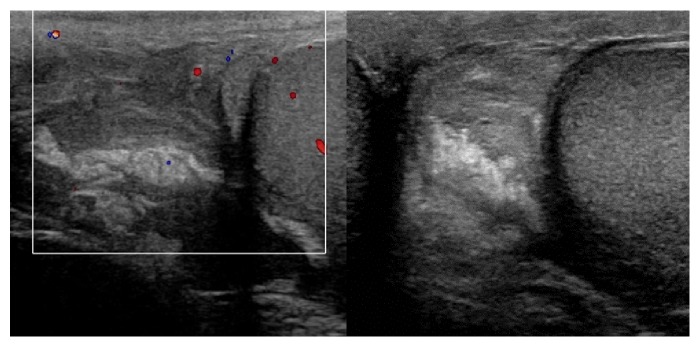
Doppler ultrasonography: a complex collection with internal echoes which is avascular on color Doppler, compatible with hematoma of the spermatic cord.
